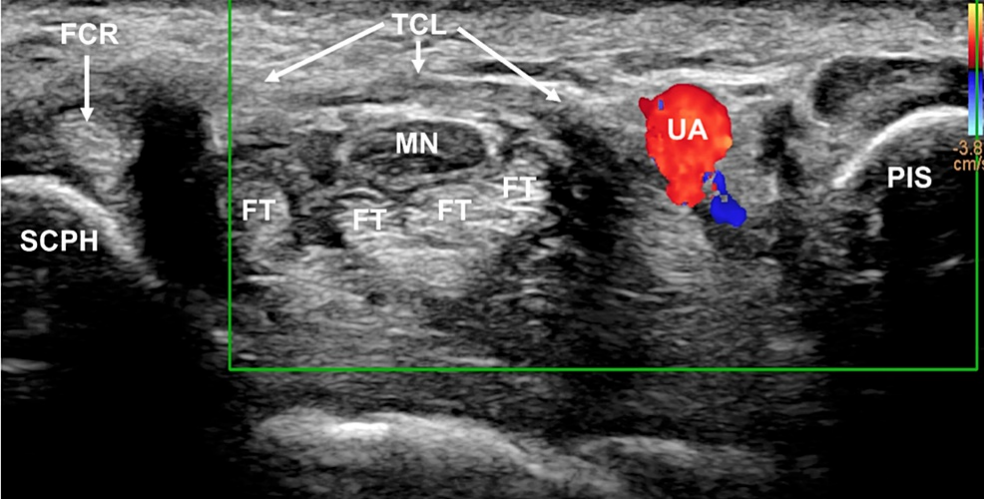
Correlative ultrasound image obtained with a 4-18MHz transducer in a live patient All anatomic structures seen in Figure 2 are visualized, demonstrating the wide field of view of ultrasound. This image was obtained with the Doppler ultrasound function being activated, which clearly identifies the pulsating ulnar artery. Label and orientation as the same as in Figure 2. Figure property of the authors
